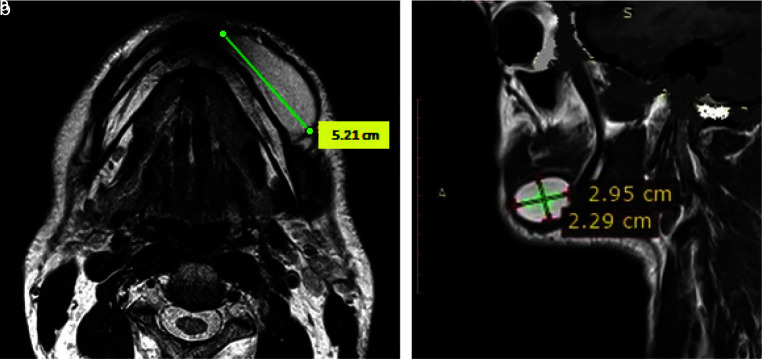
Pre-operative Magnetic Resonance Imaging showing a well-defined hyper dense lesion in the mandibular vestibule. a: Axial view, b: Sagittal view
